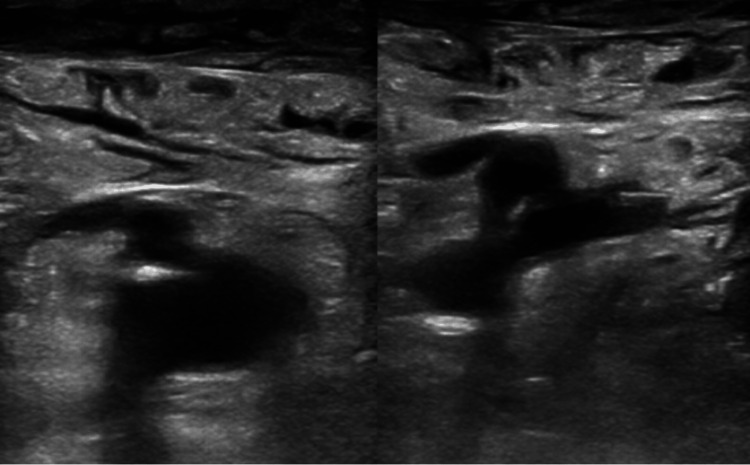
Venous Doppler lower extremities showing no deep vein thrombosis
